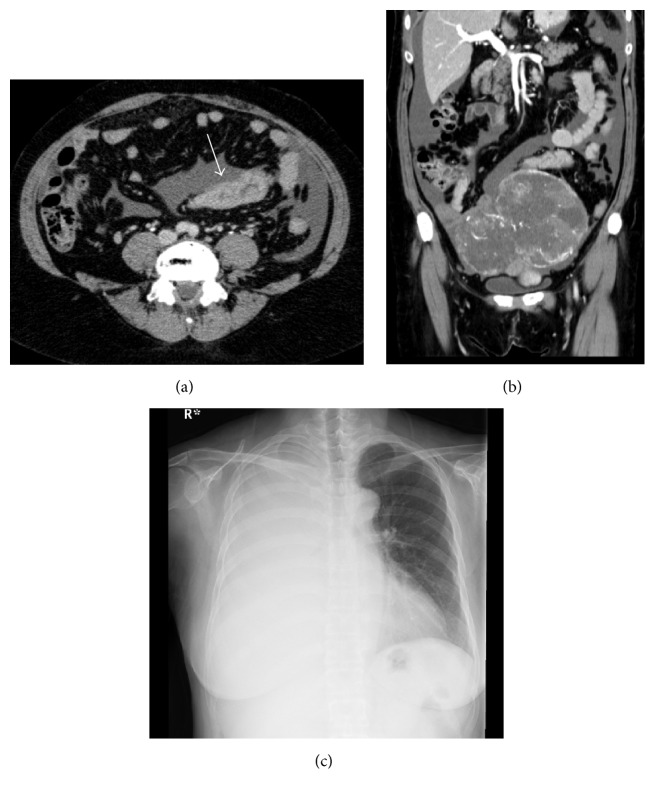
Case  1. Contrast-enhanced CT scan shows wall thickening of the sigmoid colon with enhancement (arrow; (a) axial plane) and a well-circumscribed and heterogeneously enhanced tumor of the ovary ((b) coronal plane). Chest X-ray shows a massive pleural effusion in right thorax (c).
